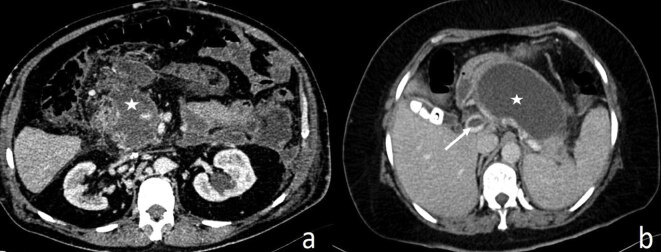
Walled-off necrosis (asterisk) surrounding portal vein (a) and walled-off necrosis (asterisk) reaching out into the portal vein (arrow) (b) are seen on the axial CT images of two different patients.
